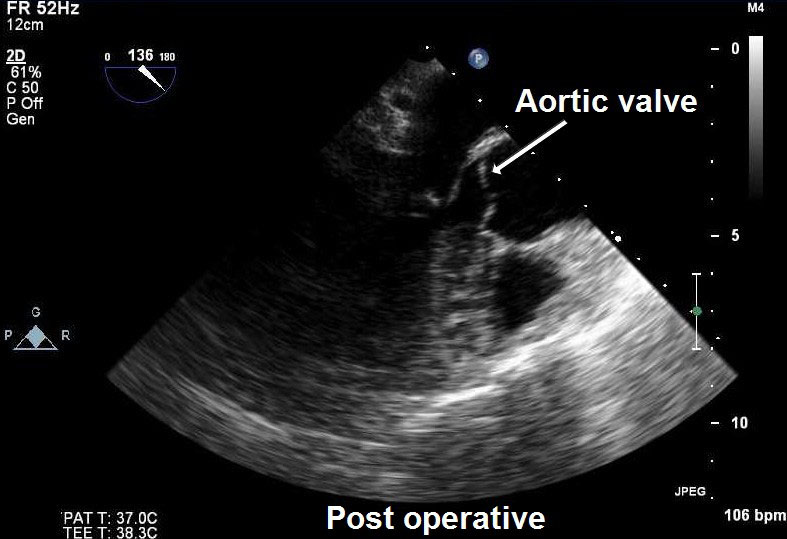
Postoperative trans-oesophageal echocardiography confirming complete resection of the tumour and normal aortic valve (ME AV long-axis view).
